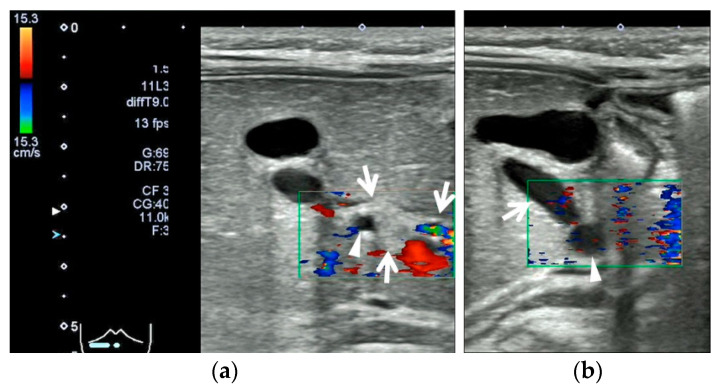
Abdominal ultrasonographic findings in Case 2 after 3 h of fasting; Aplio 300 (Cannon Medical Systems), linear probe, B-mode frequency: 9.0 MHz, pulse repetition frequency: 12 fps. (a) Transverse section in the right upper abdominal region: A common hepatic duct (arrowhead) was seen within a hyperechoic portal mass (enclosed by arrows). (b) Longitudinal section in the right upper abdominal region: The gallbladder lumen was open, and the gallbladder wall was normal in appearance (1.5 mm thick with a two-layered appearance). The choledochal duct was open (arrow), and the common hepatic duct (arrowhead) was tapered toward the hilar region. The maximum diameter and cross length of the gallbladder lumen were 11 × 11 mm.
